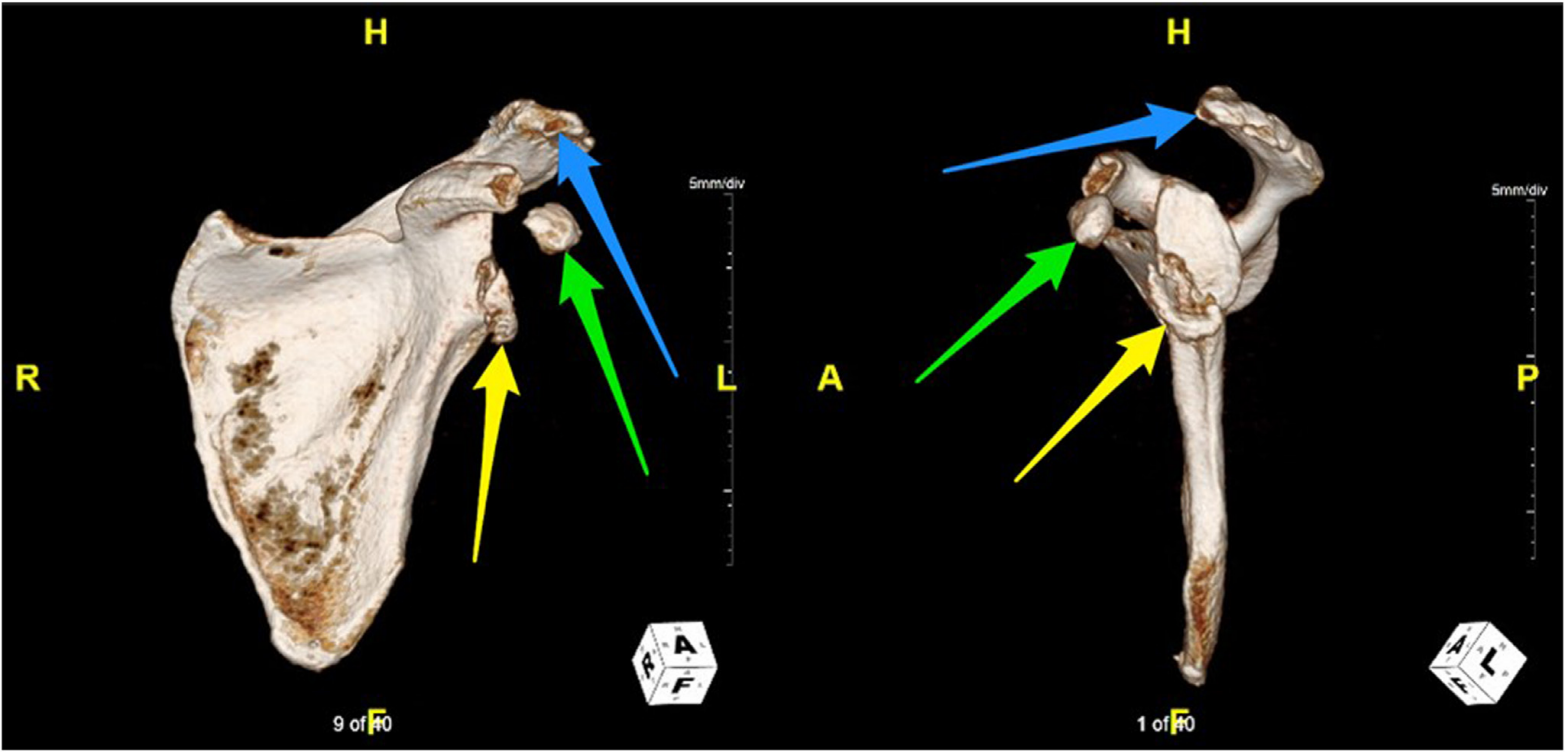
Three-dimensional computed tomography reconstruction (2 views) following orthopedic surgery consultation. *Yellow arrows* indicate bony Bankart lesion, *green arrows* indicate coracoid avulsion, and *blue arrows* indicate likely site of acromial tip avulsion.
